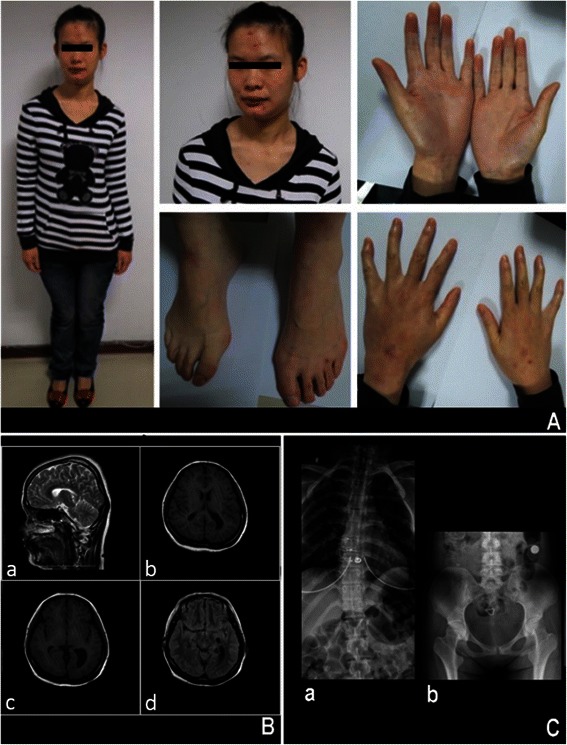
Clinical and iconography description of the patient. A) Present patients appearance and facial characteristics. B) Brain MRI images show brain structural abnormity: (a) T1-weighed sagittal section through the midline, showing corpus callosum hypoplasia; (b) T2-weighed axial section showing colpocephaly; (c) T2-weighed axial section showing asymmetric lateral ventricles; (d) T2-weighed axial section showing choroid fissure cysts on the left. C) Radiographic analysis show skeletal structure abnormity: (a) Thoracic vertebra showed T8-T10 scoliosis rightward; (b) pelvic obliquity.
